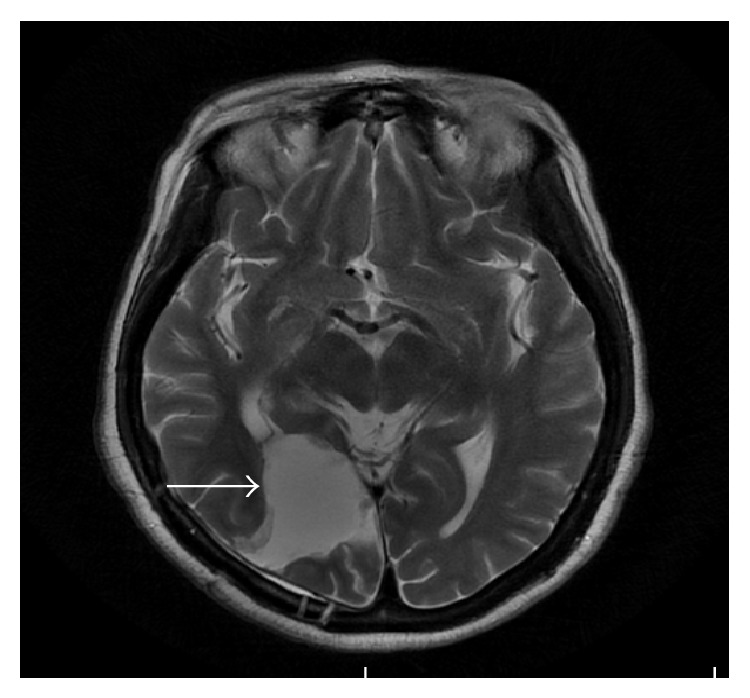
T2-weighted cranial MRI showed a 4.5 × 4.1 × 6.2-cm solitary BM (white arrow) in the right parietal-temporal-occipital lobe with intense contrast enhancement and a 0.8 cm displacement of midline structures. The lesion also compresses occipital horn of the lateral ventricle posteriorly and is surrounded by a wide area with alteration of signal that could correspond to edema or tumor infiltration.
